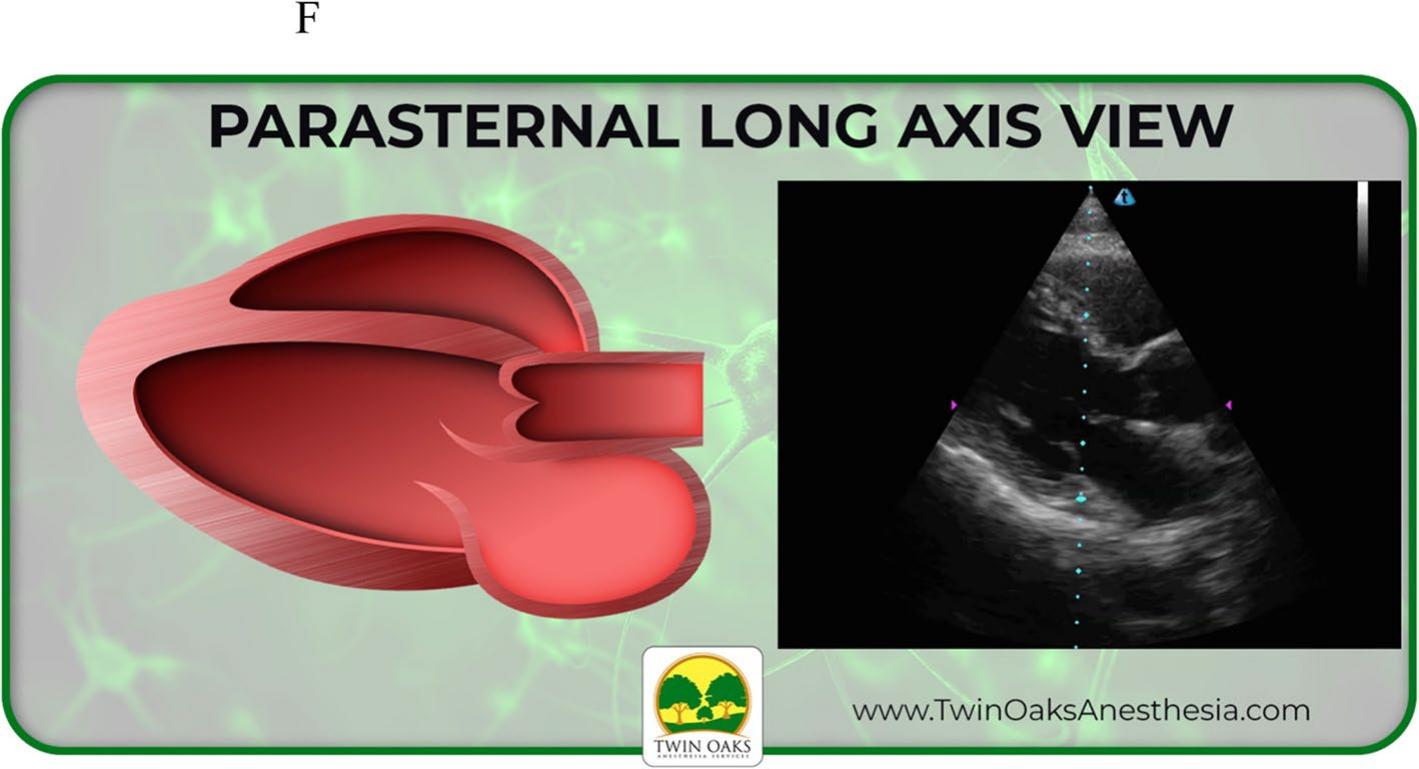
Study graphic of parasternal long axis graphic and corresponding TTE view
